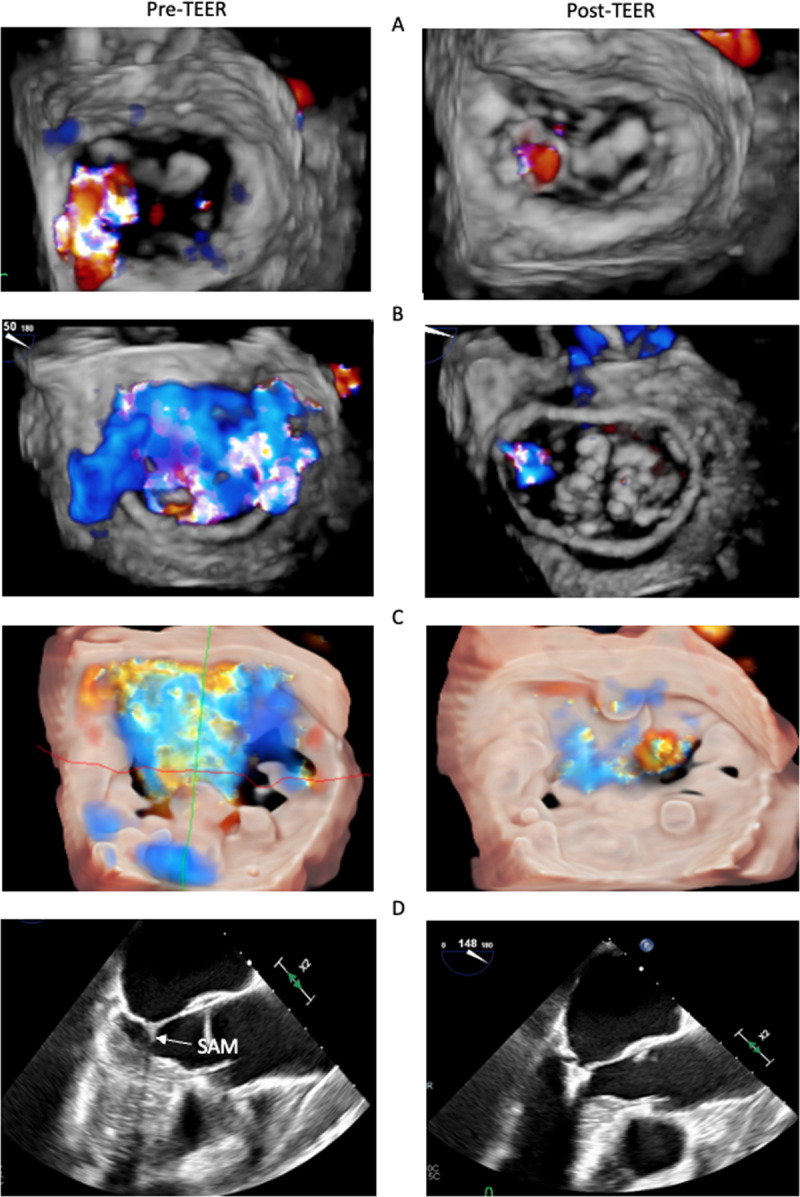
Pre- and post-transcatheter edge-to-edge repair (TEER) echocardiography in challenging cases of TEER in (A) commissural mitral regurgitation; (B) prior ring annuloplasty; (C) severe mitral annular calcification; and (D) hypertrophic cardiomyopathy with left ventricular outflow tract obstruction due to systolic anterior motion (SAM).
